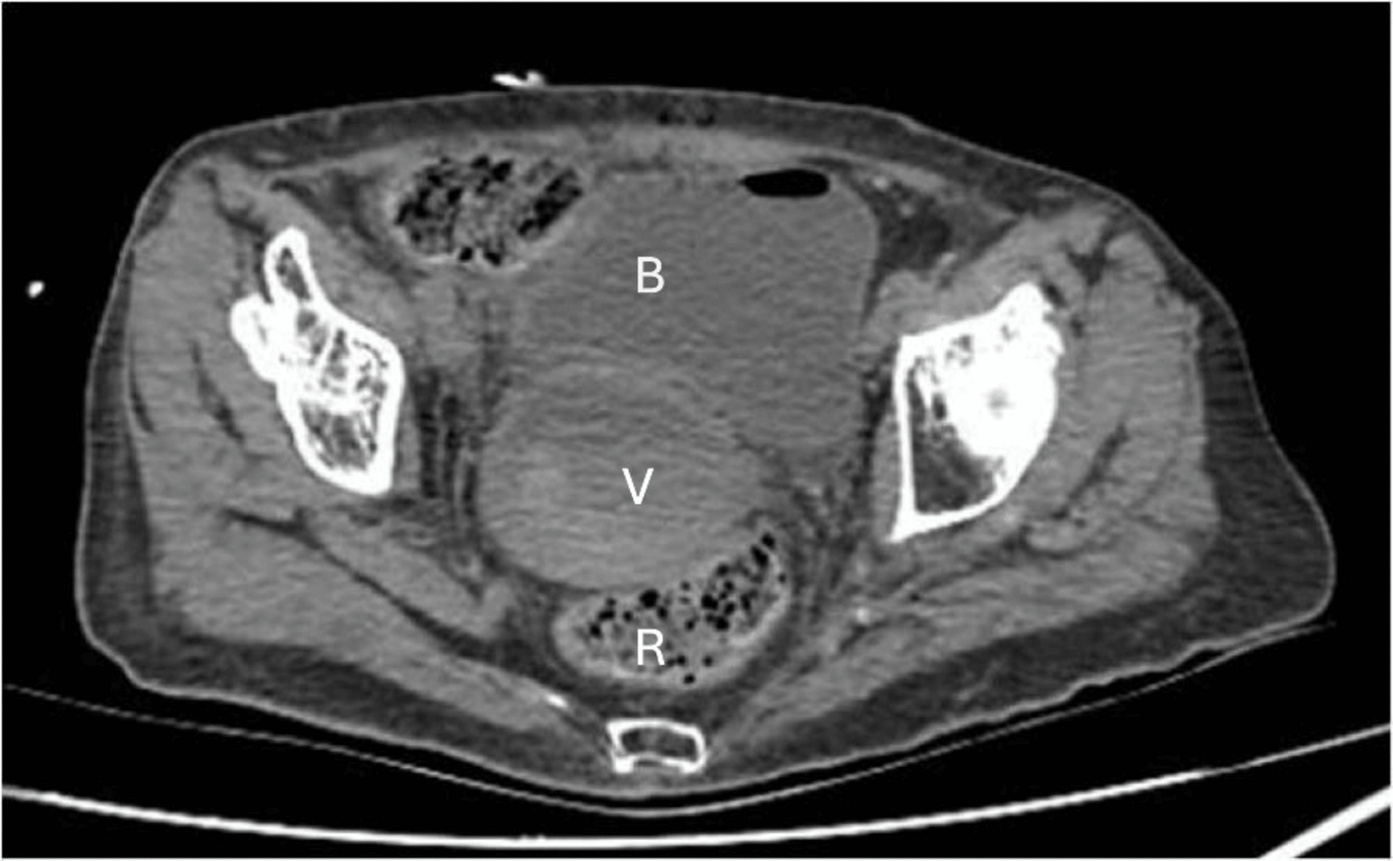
CT findings CT showing the fluid-filled vaginal cavity (V) with high-signal intensity, supporting the diagnosis of hydro-pyo-hematocolpos CT: computed tomography; B: bladder; R: rectum; V: vaginal cavity
